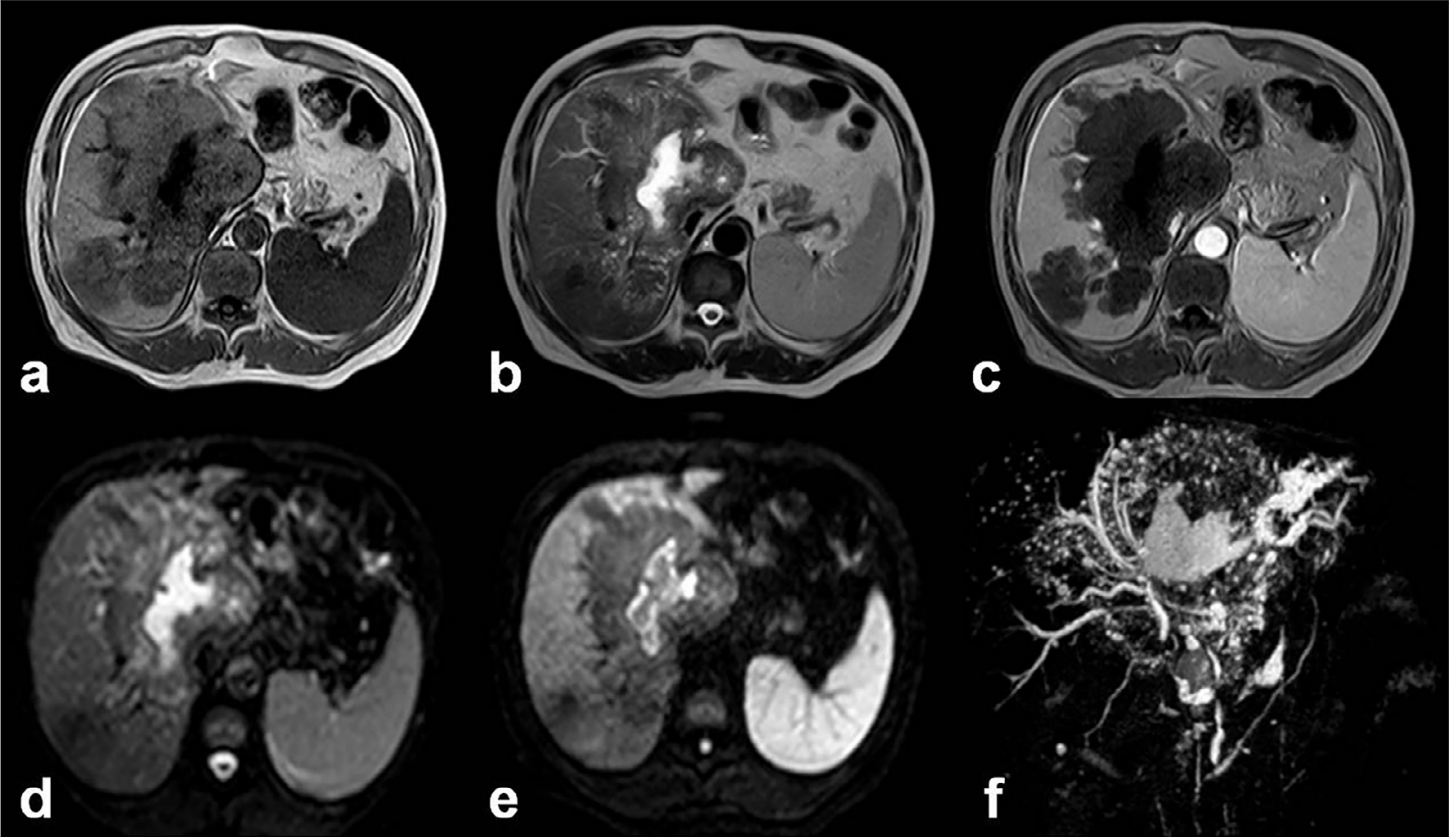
Alveolar echinococcosis in a 55-year-old man.* (a) Axial unenhanced T1-weighted Magnetic Resonance (MR) image shows infiltrating diffused mass with hypo-intense signal in the right and left lobes of the liver. (b) Axial unenhanced T2-weighted MR image shows heterogeneous signal. The high signal intensity corresponds to small cystic and necrotic components of mass lesion. (c) Axial enhanced T1-weighted image with gadolinium shows no enhancement in the mass lesion. (d, e) Diffusion-weighted MR images obtained with b value of 0 s/mm^2^ and 600 s/mm^2^. Hypo-intense signal characterizes the cystic and necrotic components of the lesion whereas hyper-intense signal seen in the central necrotic part reveals restricted diffusion due to bacterial superinfection. (f) MR Cholangiopancreatography of the liver shows several small cystic and necrotic components of the mass lesion with dilated right hepatic duct, constricted upper part of common bile duct, and mildly dilated pancreatic duct.
